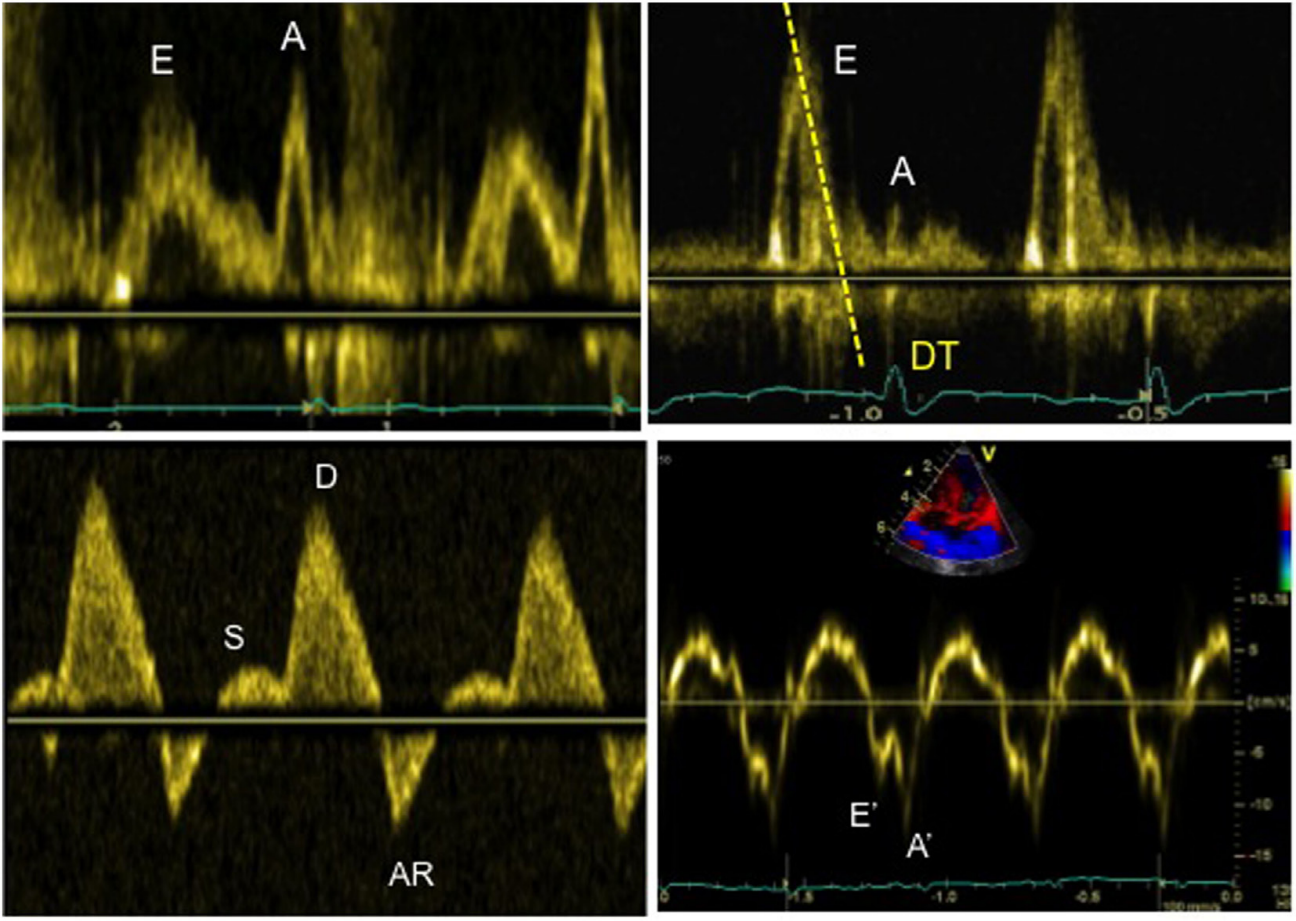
Evaluation of diastolic function in a patient with severe diastolic dysfunction: (1) mitral inflow Doppler pattern with E and A wave reversal (above left image); (2) mitral inflow Doppler pattern with sharp E wave DT (above right image); (3) pulmonary venous Doppler with prominent diastolic flow, S/D reversal and increased velocity of AR wave (below left image); (4) TDI at the mitral annulus with E′/A′ reversal and decreased E′ wave velocity <8 cm/s (below right image).
